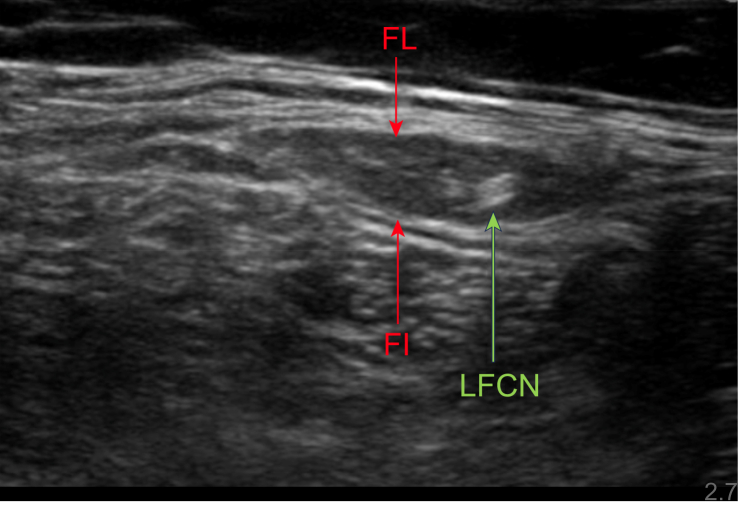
Ultrasound image showing the lateral femoral cutaneous nerve (LFCN) located between fascia lata (FL) and fascia iliaca (FI).
